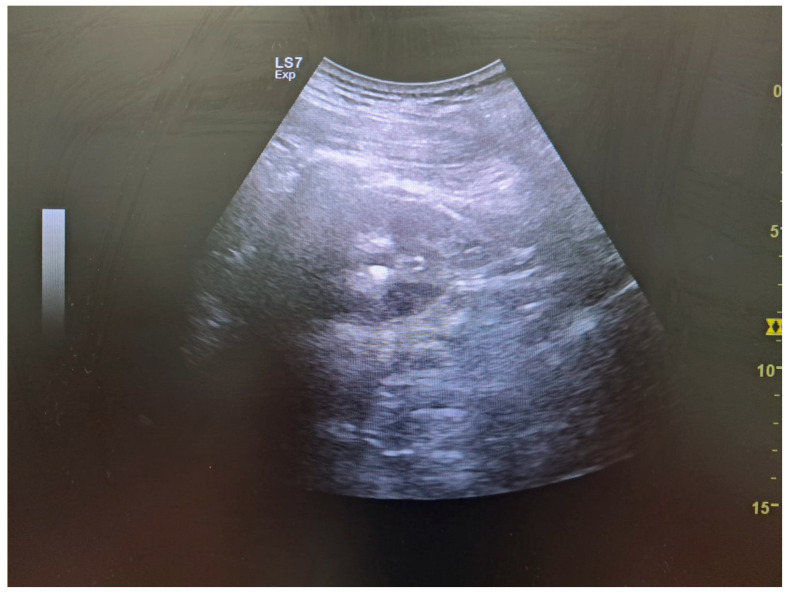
Kidney stone and acoustic shadow behind it (ultrasonography).
